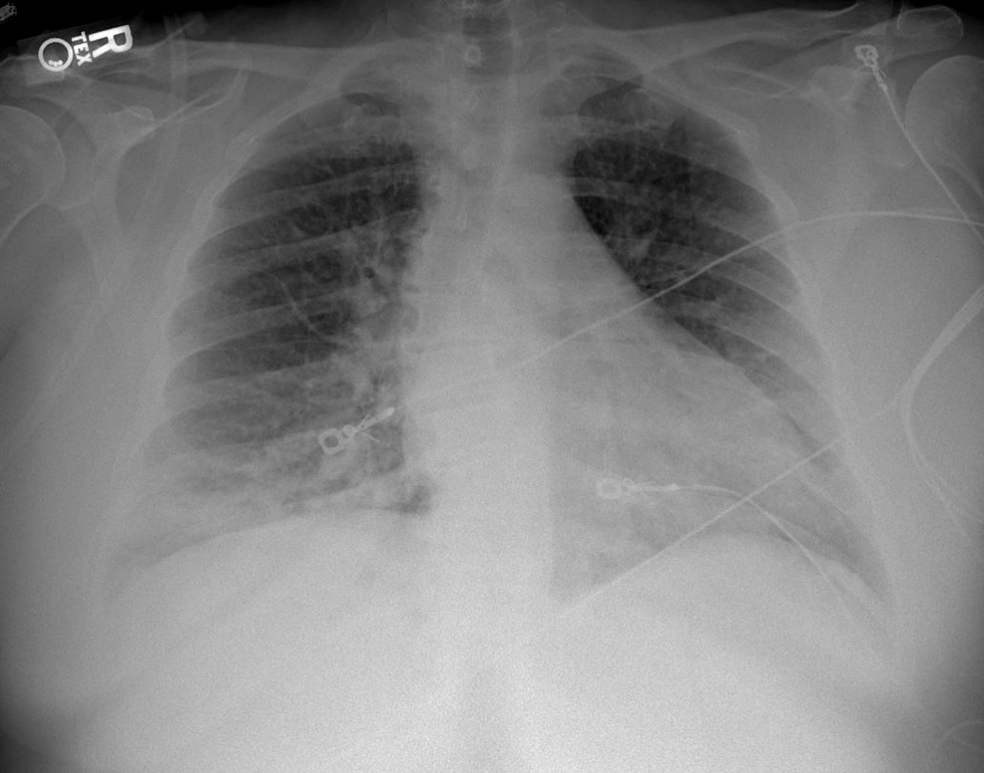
Chest X-ray. Chest X-ray revealed patchy airspace opacities mainly in the right middle and lower zones.
